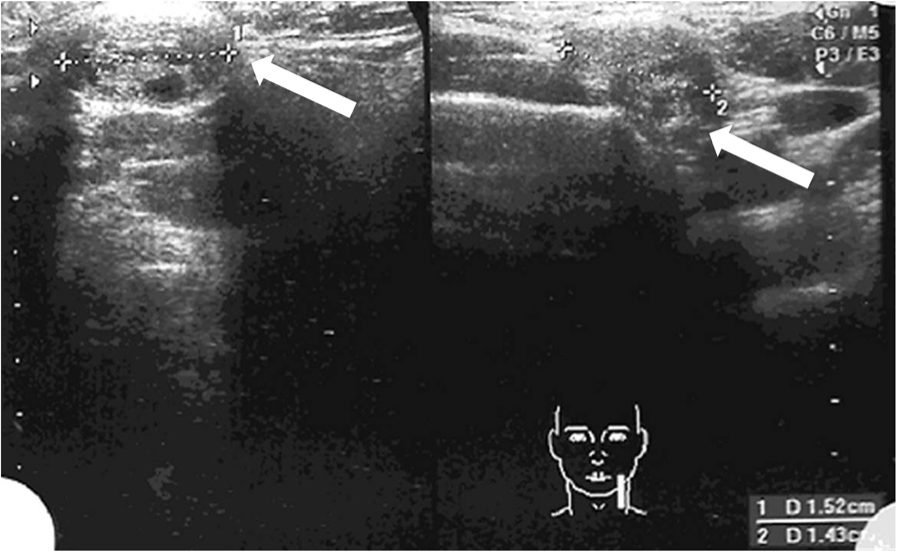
Neck ultrasonography. The arrows show two submandibular nodes with thyroid tissue.
